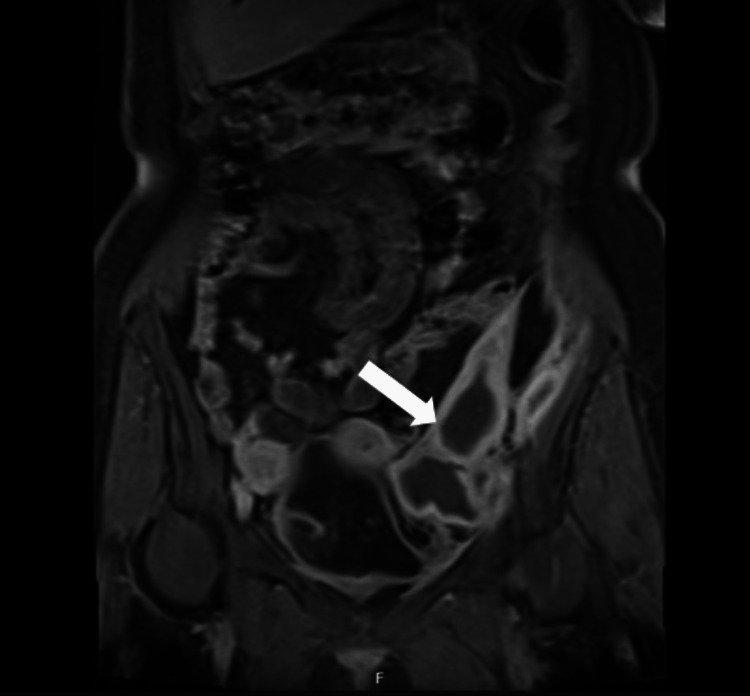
Magnetic resonance imaging - Fat-suppressed contrast-enhanced T1-weighted image in the coronal plane showing a multiloculated hematoma along the left iliac muscle and in the left iliac fossa. Arrow indicates the hematoma
